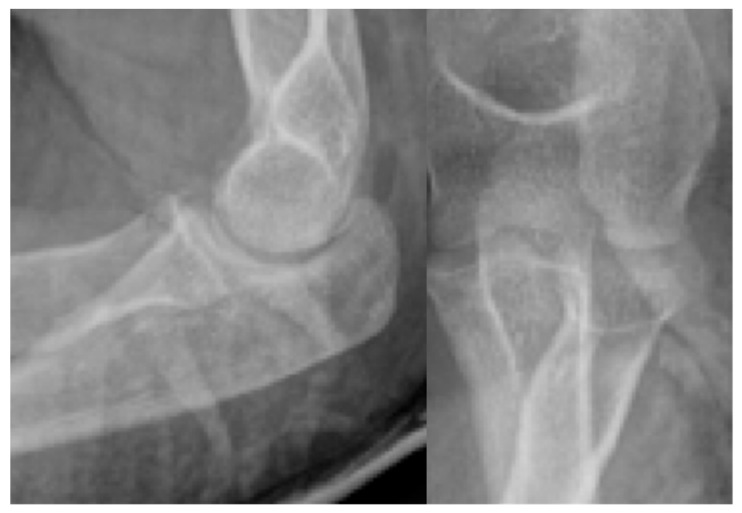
Postoperative X-ray showing the perfect position of the radial head.
